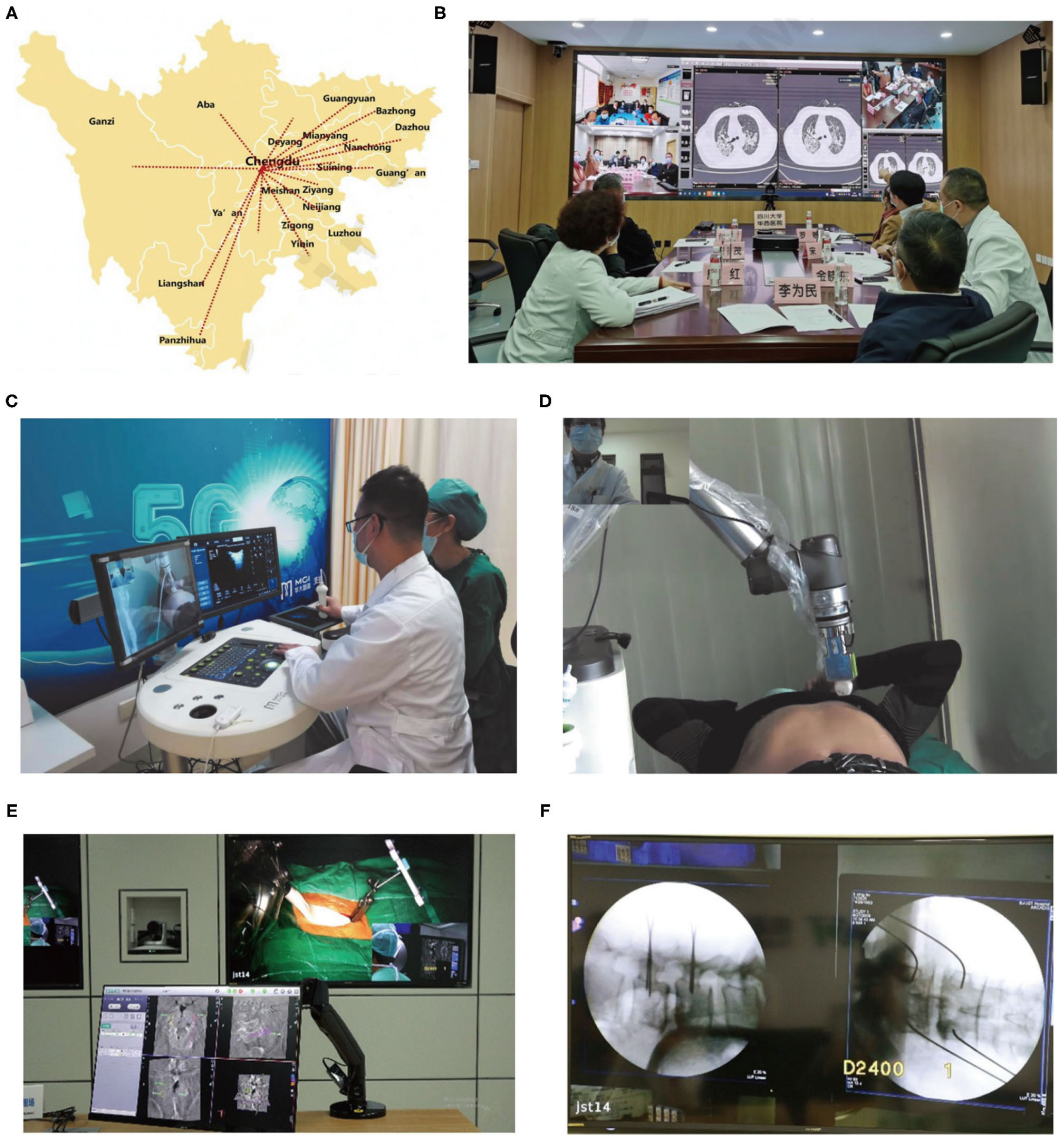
Telemedicine based on fifth-generation (5G) network and robotic technology for disease diagnosis and treatment during the COVID-19 outbreak. (A) 5G telemedicine platform of Sichuan Province of China developed during this pandemic. (B) The web-based real-time video tele-consultation provided by a multidisciplinary medical team based on “5G Dual Gigabit network” to deal with cases vulnerable to severe COVID-19 in western China (57). (C,D) 5G remote robotic ultrasound diagnostic system used in Fangcang shelter hospitals (82). (E) 5G network-based tele-ultrasound system tele-robotic spinal surgery including screw planning at master control room and (F) K-wire placement (63).
